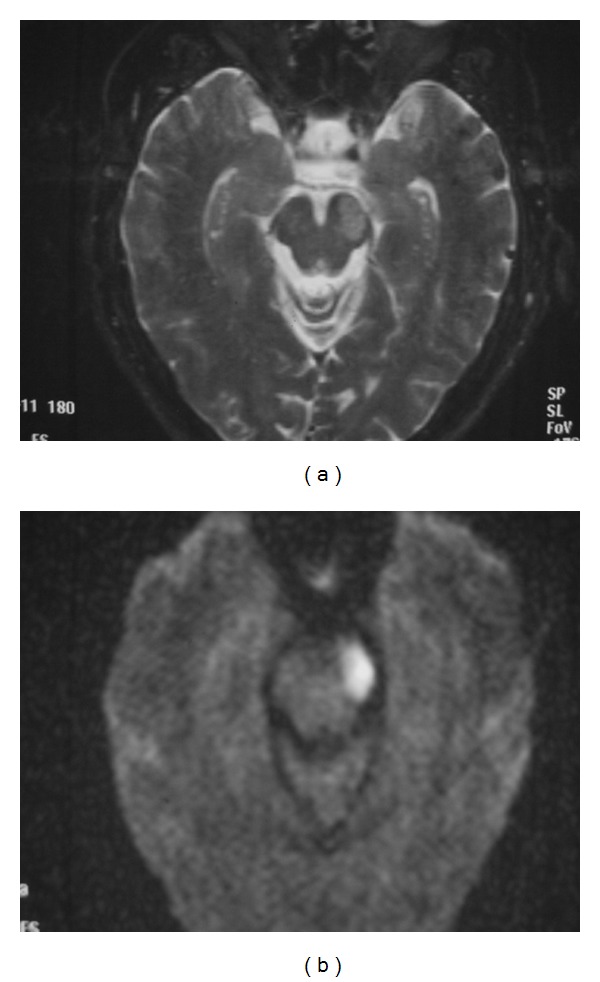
Infarct of the crus cerebri. Axial T2 (a), and DWI (b) MR images of the midbrain with subacute infarction involving the left cerebral peduncle. This patient presented with right-sided face, arm and leg weakness due to involvement of the corticobulbar and corticospinal tracts within the left cerebral peduncle.
